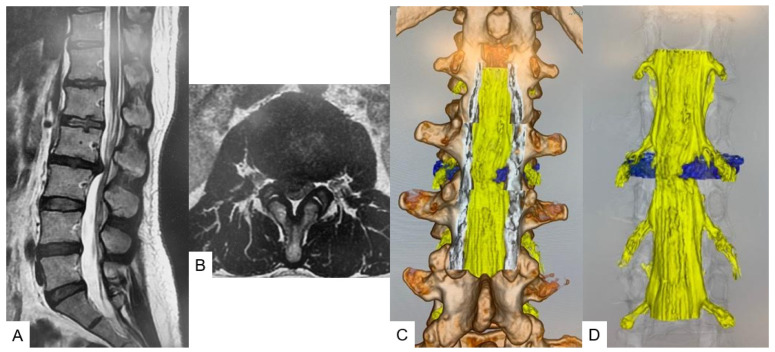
A 44-year-old female, right L2/3 paramedial disc herniation. (A): T2 weighted midsagittal MRI. (B): L2/3 T2 weighted axial MRI, (C): Posterior view. (D): Without bony structure.
